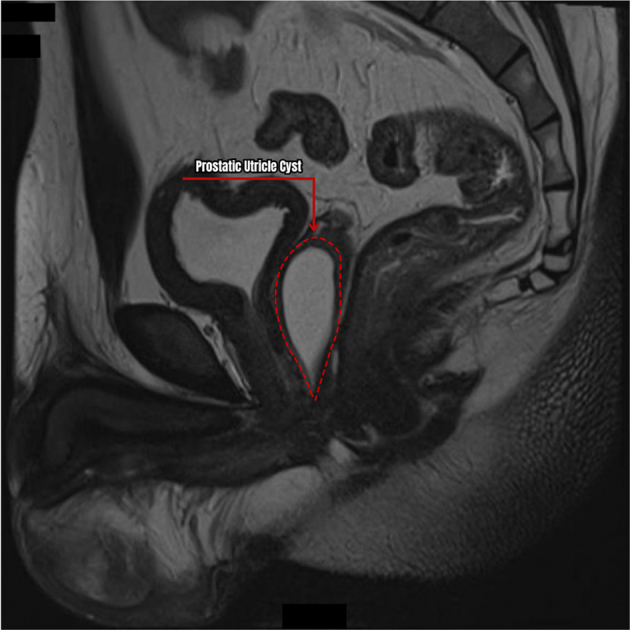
MRI T2-weighted sagittal view of the large prostatic utricle cyst showing its relationship to the rectum and urinary bladder.
